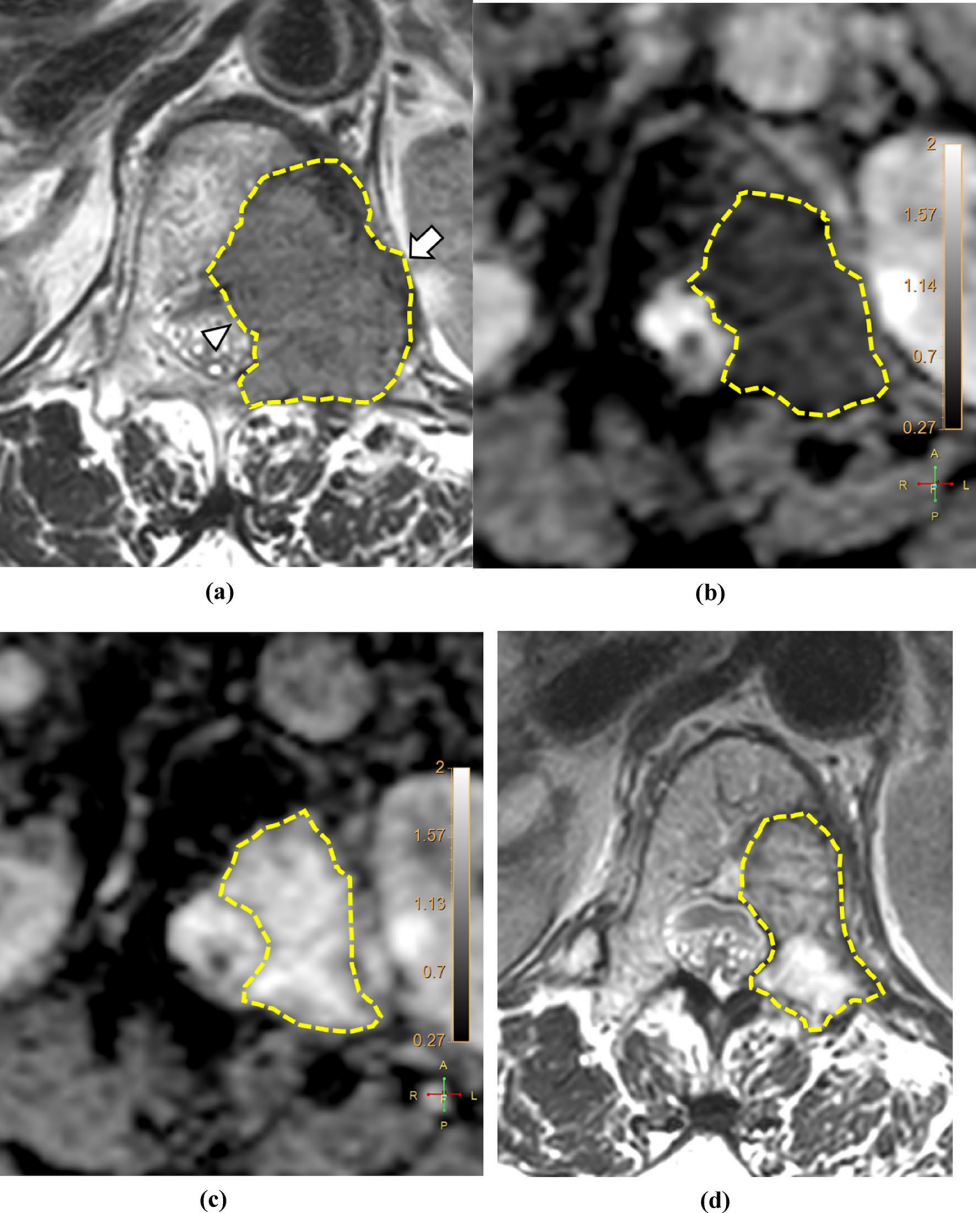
Images of a 71-year-old woman with metastasis to the T12 vertebra from a hepatocellular carcinoma. The tumor margin is delineated by yellow dotted lines. (a) The T2WI of the baseline MRI shows a 2.6 × 3.7-cm-sized metastatic lesion with an extraosseous extension to the left paravertebral (arrow) and epidural spaces (arrowhead). Compared with (b) the baseline MRI, the average mean ADC value measured by readers I and II in the (c) first post-RT MRI increased from 0.68 to 1.39 (× 10^–3^ mm^2^/s). (d) The T2WI of the second post-RT MRI shows that the size of the lesion decreased to 1.6 × 3.4 cm, representing a partial response. *ADC* apparent diffusion coefficient, *MRI* magnetic resonance imaging, *RT* radiation therapy, *T2WI* T2-weighted image.
